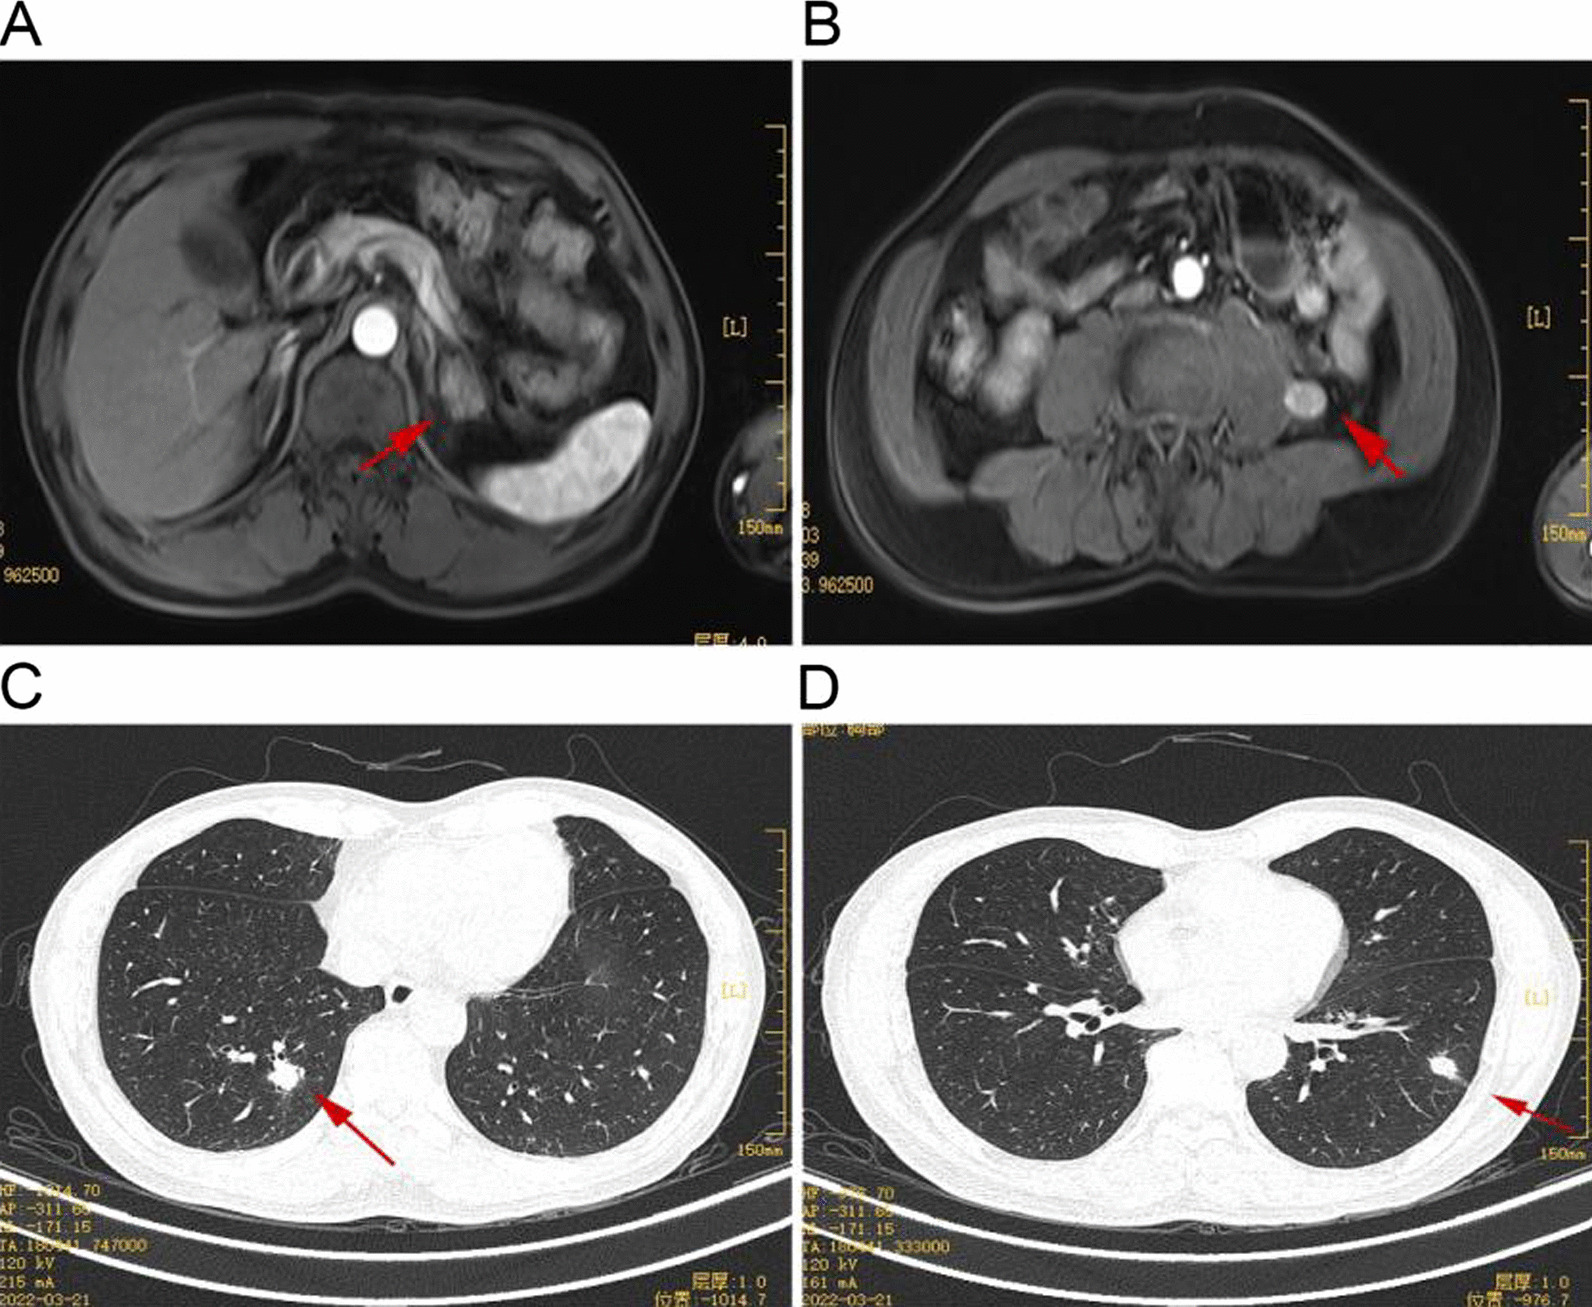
A, B Abdominal MRI images show a mass in the left adrenal region and a mass beside the left psoas muscle, considered a local tumor recurrence. C, D The chest CT investigations show multiple nodule shadows in both lungs, considering distant metastasis of the tumor
